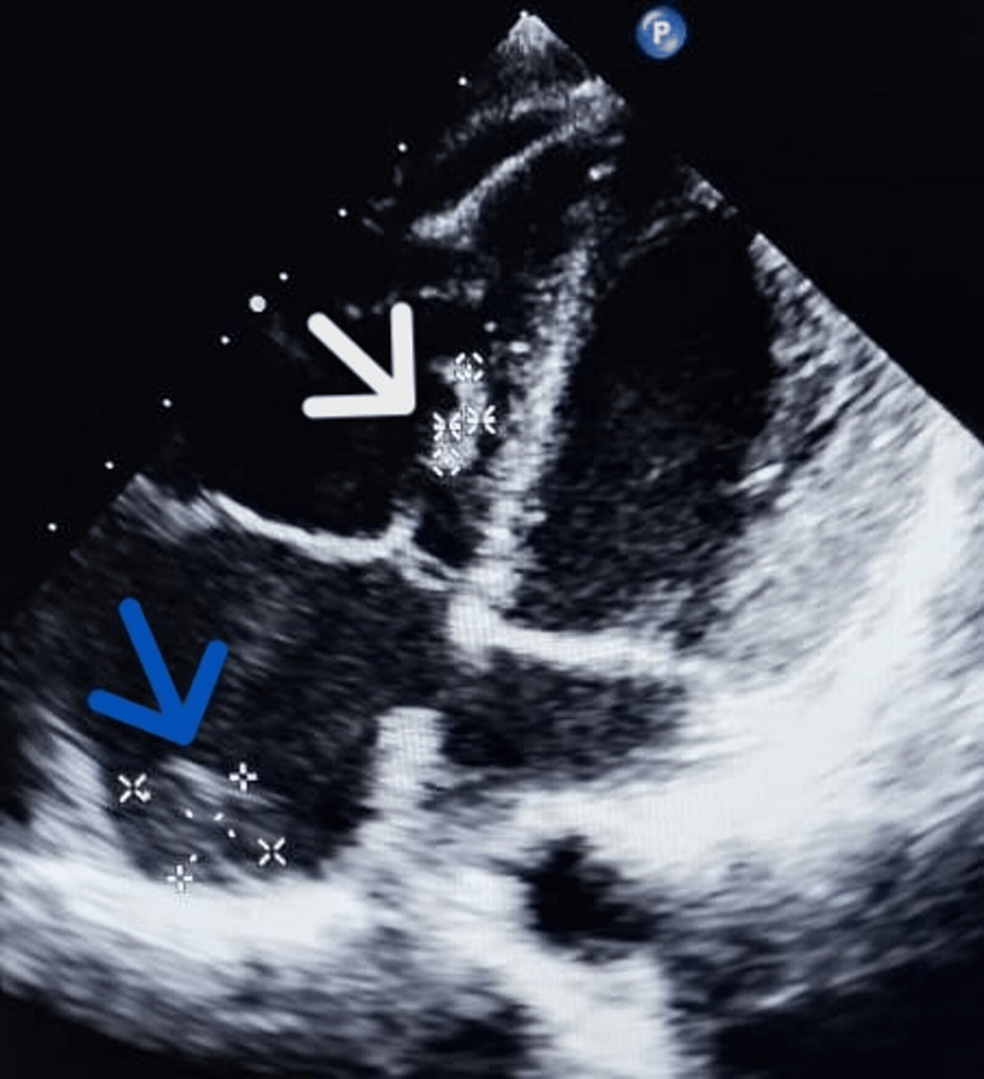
Transthoracic echocardiography, in apical four-chamber view, demonstrating a right ventricular thrombus 7×15 mm (white arrow), and a large right atrium thrombus 14×18 mm (blue arrow)
